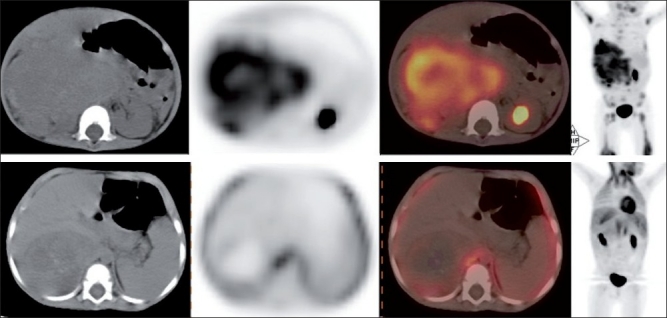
Transaxial CT, PET, PET-CT and maximum intensity projection images (L-R) images of a patient with NB. Baseline scan (upper row) shows a right suprarenal mass with intense uptake. Scan done post two cycles of NACT (lower row) shows reduction in the size of the mass as well as FDG uptake suggestive treatment response in CT and PET-CT
